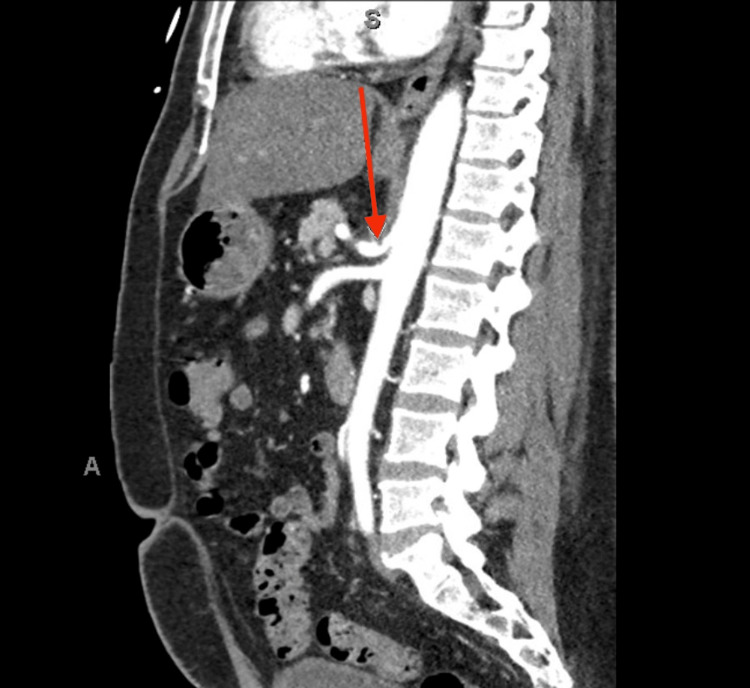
Computed tomography (CT) angiogram findings The CT angiogram revealed anterior superior indentation of the proximal celiac artery by the diaphragmatic crus with a J-shaped configuration and post-stenotic dilation (red arrow), consistent with MALS. MALS: median arcuate ligament syndrome
